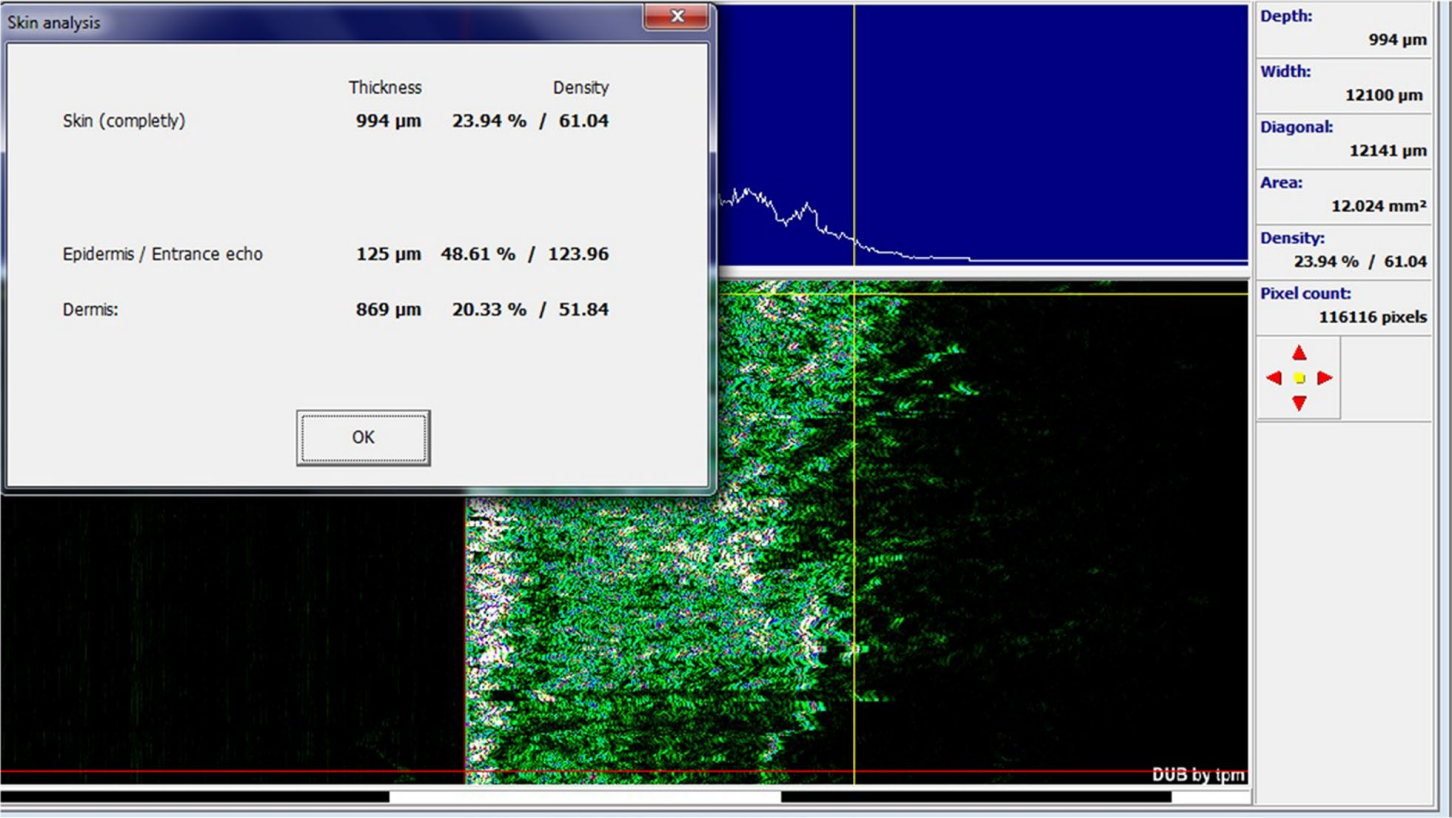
Sonographic findings before intervention with fractional CO_2_ laser
